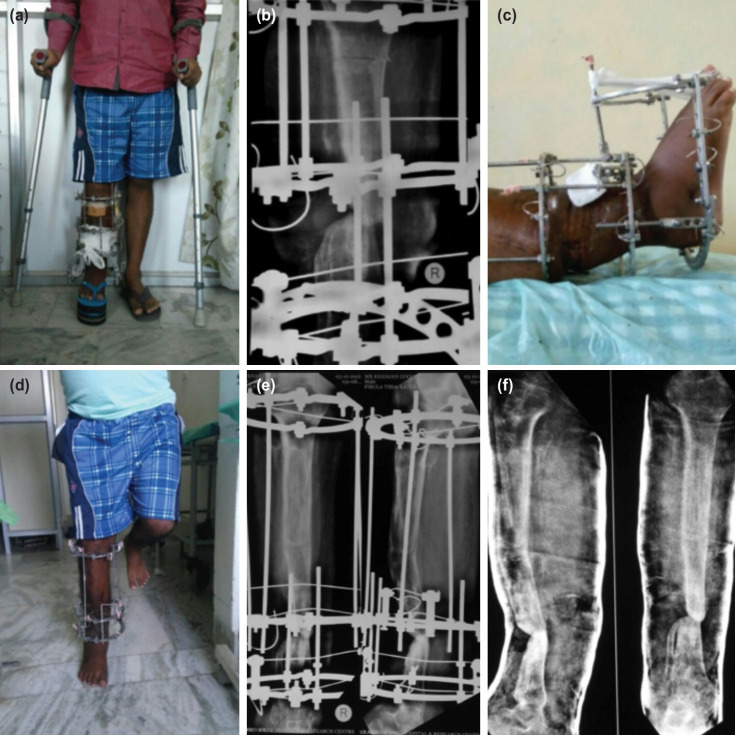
(a) Clinical picture of a patient treated with ASRL. (b) Post-op Radiograph showing docking site and corticotomy being done. (c) Clinical picture showing docking site with foot-frame. (d) Clinical picture during follow-up (e) Radiographs at follow-up showing regenerate and docking site union. (f) Radiographs after Ring removal and Cast immobilisation.
